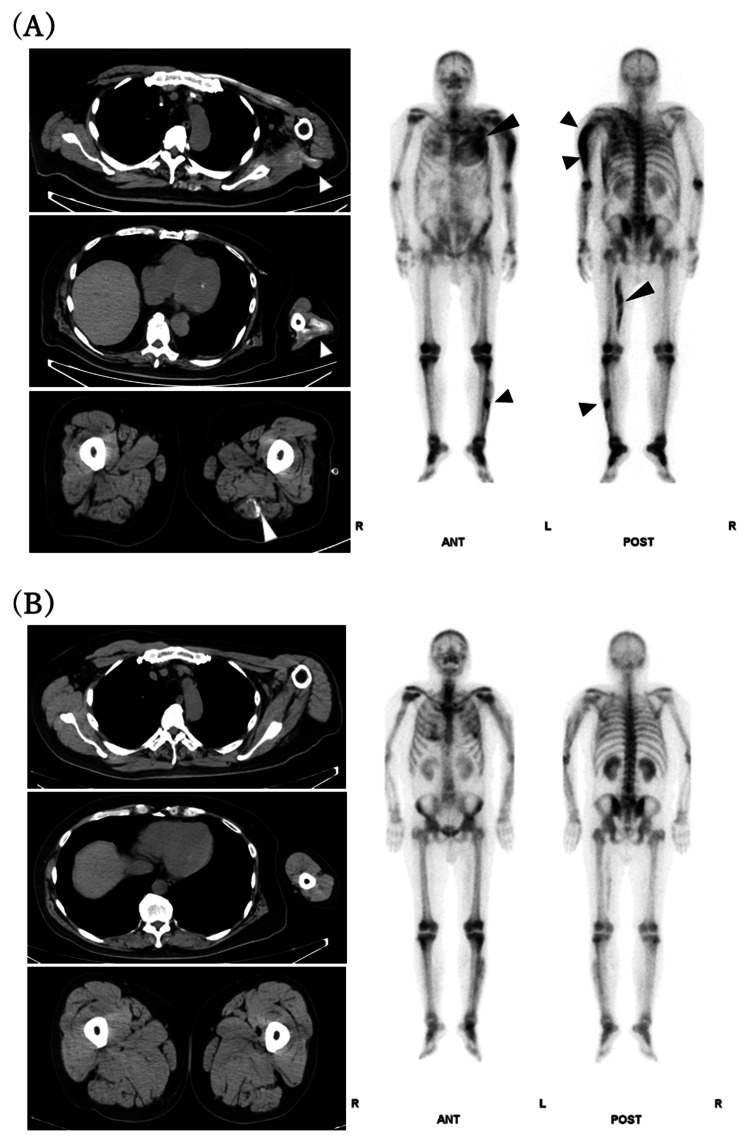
Whole-body plain CT scans and bone scintigraphy (A) At the onset of hypercalcemia (Day 26), calcium deposits (white arrowheads) are seen in the left pectoralis muscle and upper arm, as well as other muscles on the left side. Corresponding high uptake of ^99m^Tc-HMDP is observed in the same sites (black arrowheads). (B) After improvement of hypercalcemia (Day 45), these calcium deposits in the muscles have disappeared, and there is no longer any high uptake of ^99m^Tc-HMDP in those regions. ^99m^Tc-HMDP, technetium-99m hydroxymethylene diphosphonate.
